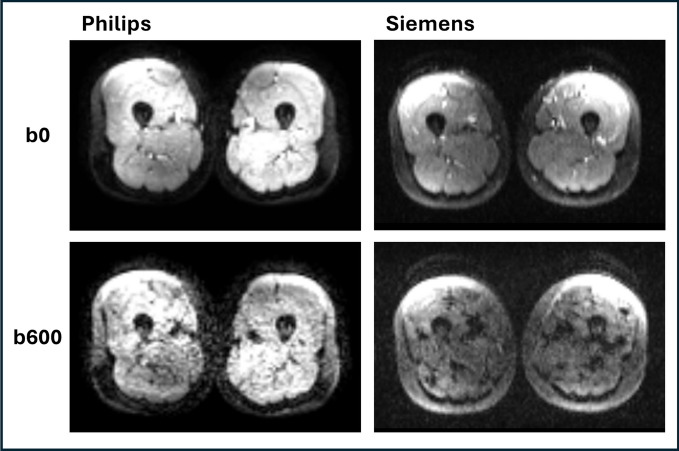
Raw diffusion-weighted images acquired on Philips and Siemens MRI systems at *b* values of 0 and 600
